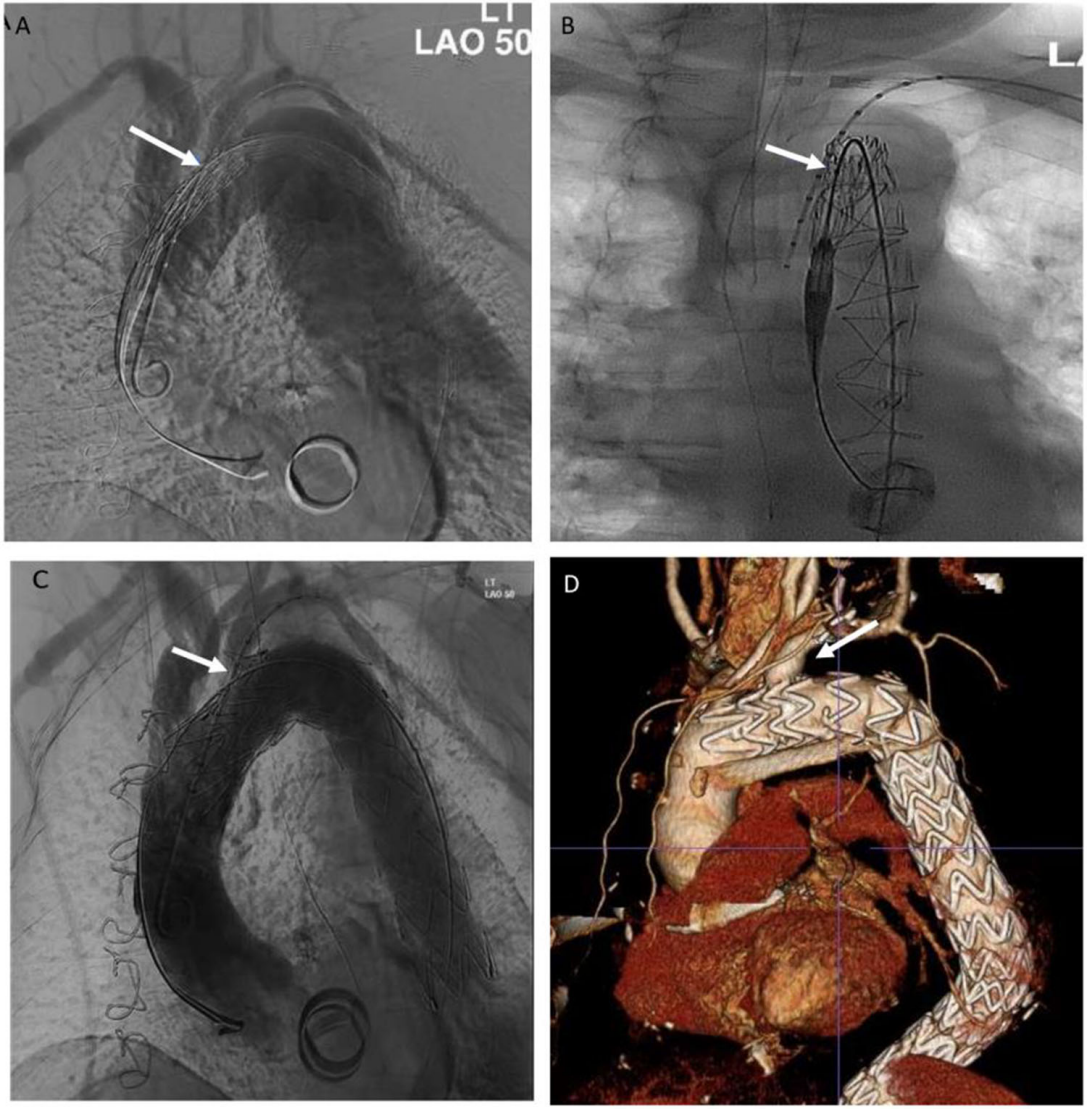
Perpendicular aortic arch angiograms showing radiopaque markers of scallop (white arrows) to left subclavian artery (LSA) with the undeployed (a, b) and deployed (c) endograft with perfusion to the LSA and no type I endoleak intraoperatively at completion angiogram (c) and at latest follow-up on computed tomography (d)
